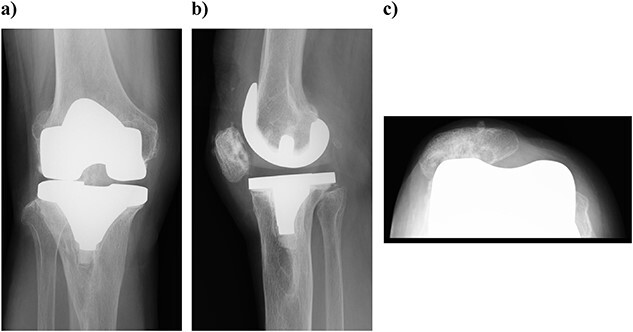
Outcome at 3 years after surgery on plain radiography: (a) standing front and (b) side. The patella is laterally subluxated in a slight valgus position.
